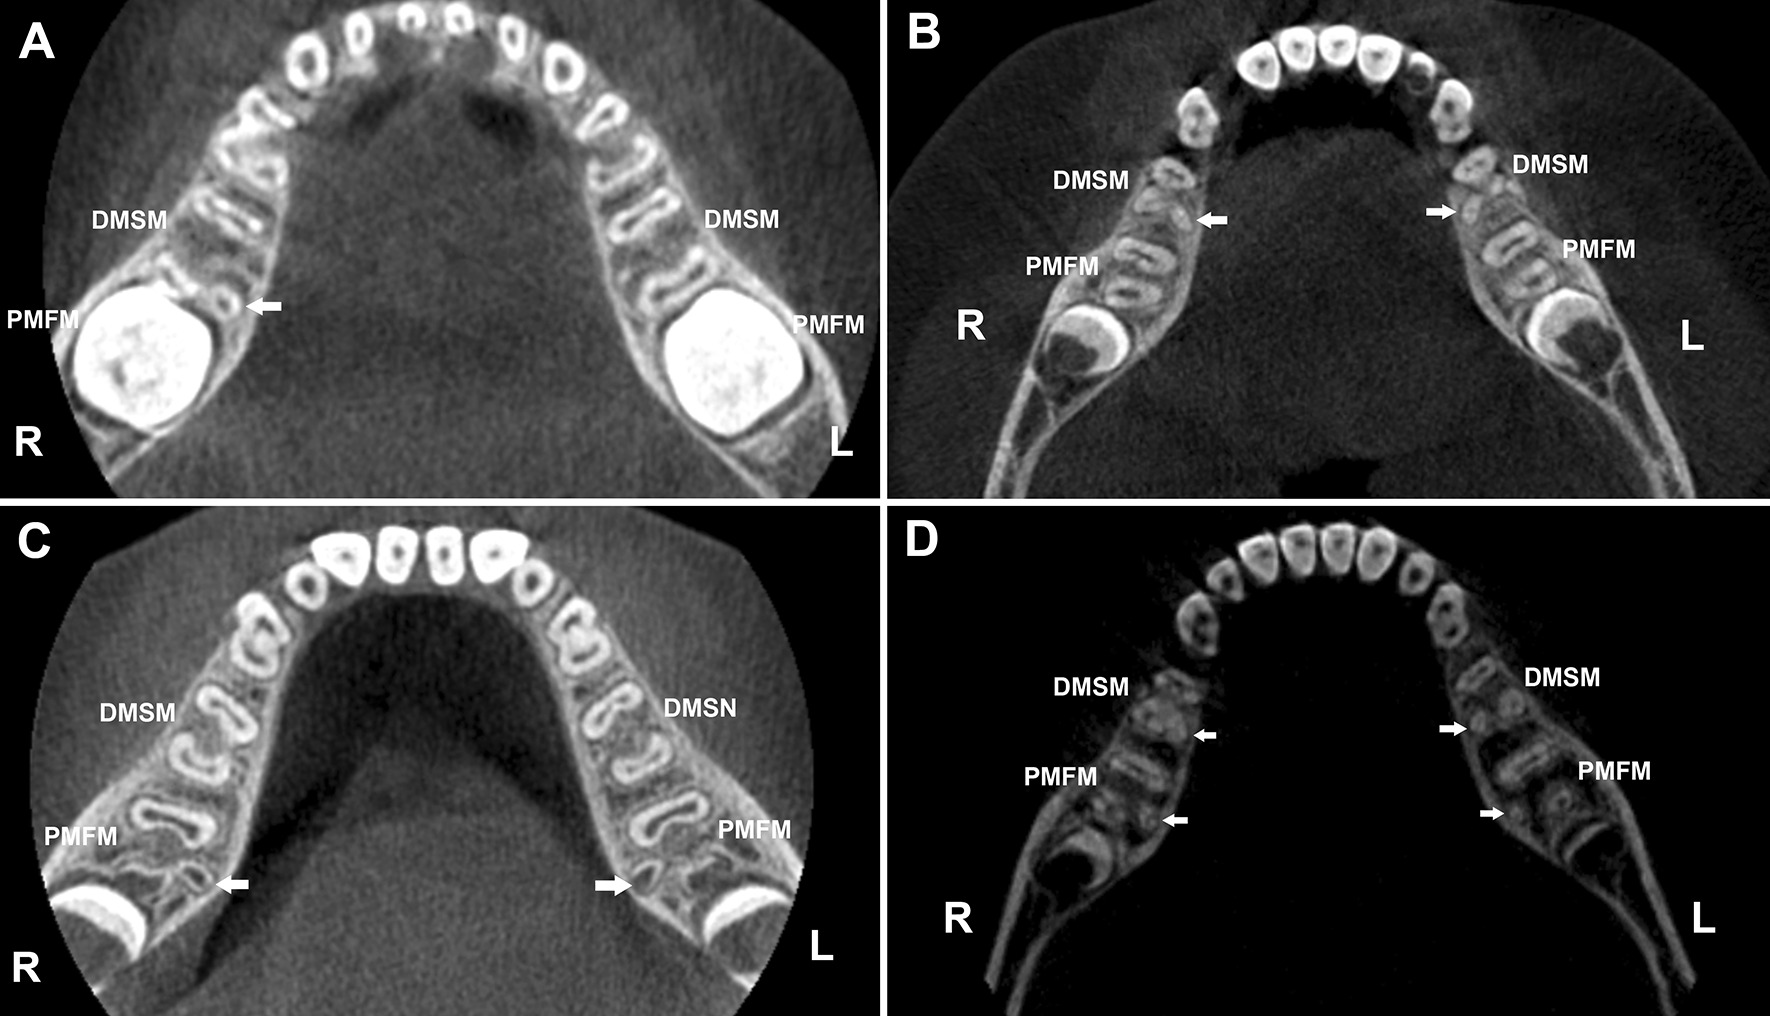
Representative CBCT images of three-rooted mandibular molars (horizontal view). a A boy aged six years exhibits a three-rooted DMSM at the right side. b A boy aged nine years exhibits bilateral three-rooted DMSMs. c A boy aged seven years exhibits bilateral three-rooted PMFMs. d A girl aged nine years exhibits bilateral three-rooted DMSMs and bilateral three-rooted PMFMs. DMSM is deciduous mandibular second molar and PMFM is permanent mandibular first molar. Arrows indicate the disto-lingual roots
